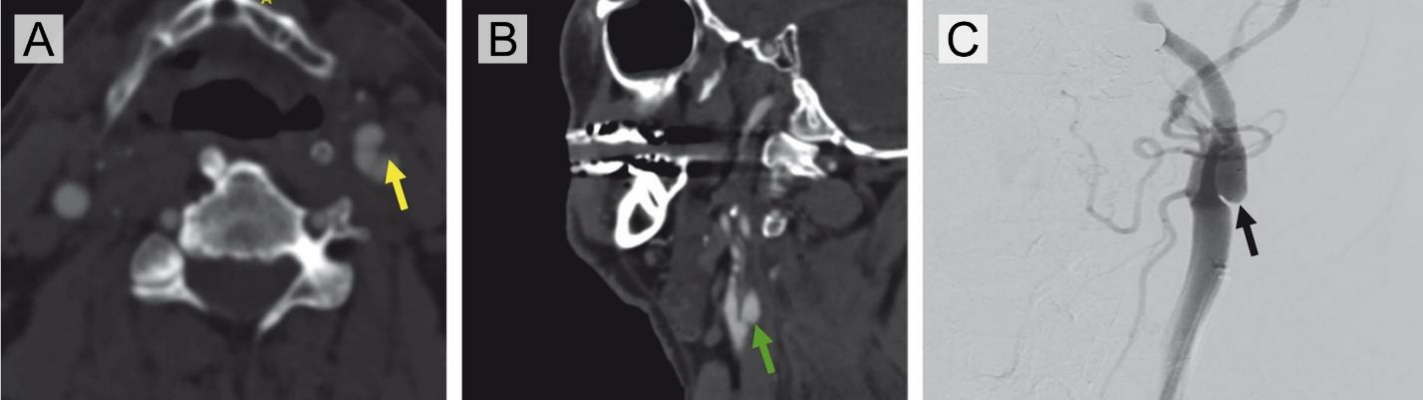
Figure 5. Radiographic imaging of a carotid artery web (CW) without evidence of superimposed thrombus in a patient with recurrent large-vessel occlusions (Case 3). (A) Axial CTA demonstrates a shelf-like projection along the posterior wall of the left internal carotid artery, consistent with a carotid web in the proximal left ICA (yellow arrow). (B) Corresponding sagittal CTA confirms the non-stenotic web morphology in the absence of atherosclerosis (green arrow). (C) Digital subtraction angiography (left common carotid contrast injection) reveals a subtle web-like filling defect in the proximal consistent with a carotid artery web (black arrow).
